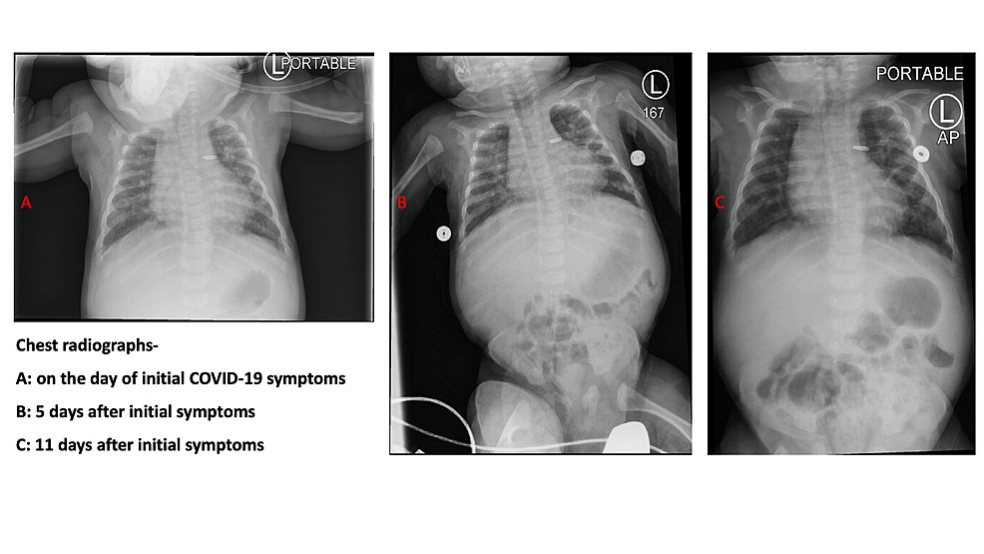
Serial chest radiographs showing the progression of pulmonary disease after diagnosis of COVID-19 Perihilar interstitial infiltrates with subsegmental atelectasis (A and B), which can be seen in viral infections. These findings were resolved over time (C). (A) On the day of initial COVID-19 symptoms, (B) five days after initial symptoms, and (C) 11 days after initial symptoms. Patent ductus arteriosus ligation clip in situ on the radiograph. COVID-19: coronavirus disease 2019, AP: anteroposterior view.
